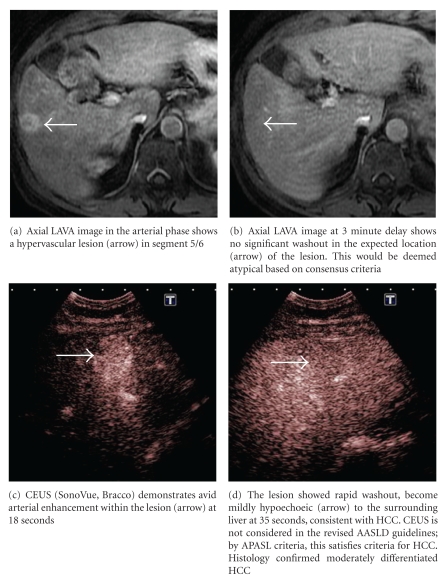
Patient with chronic HCV infection found to have a 2 cm hypoechoeic nodule on surveillence ultrasound scan. Both CEUS and multiphasic contrast-enhanced MRI were performed.
